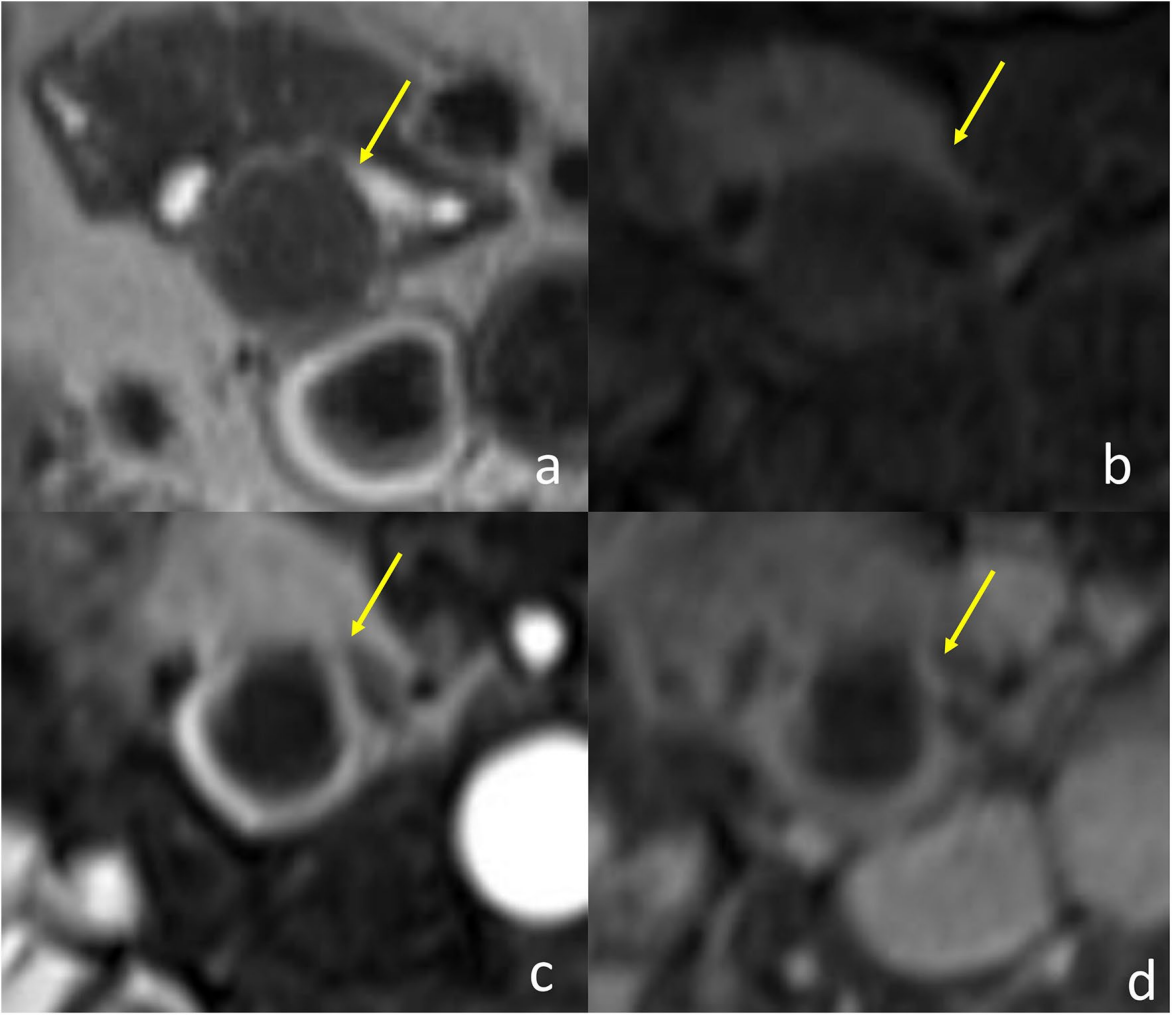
Magnetic resonance imaging (MRI). a: T2-weighted MRI showed a hypointense core region (arrow). b–d: Dynamic contrast-enhanced MRI showed a ring-shaped enhancement that appeared at the margin in early phase images and persisted until delayed phases (arrow) (b: plain phase, c: early phase, d: delayed phase)
